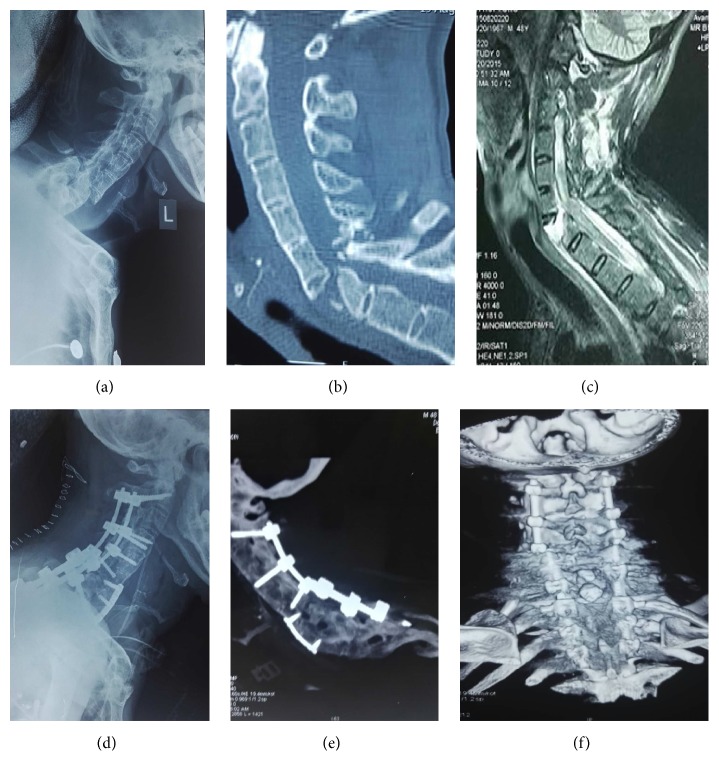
(a) A 48-year-old male was admitted to the hospital because of a car accident. The x-ray shows a typical presentation of AS. (b) Cervical CT scan showing fracture and dislocation of C6-C7, anterior defect of C7, fracture of posterior spinous process, and fracture fragments in the spinal canal. (c) MRI showing a long T2 signal in the intervertebral disc space of C6-C7 and the anterior vertebrae body, a sign of the spinal cord compression. (d, e, f) X-ray and CT scan 3 months after surgery showing good position of the internal fixation and healed fracture.
